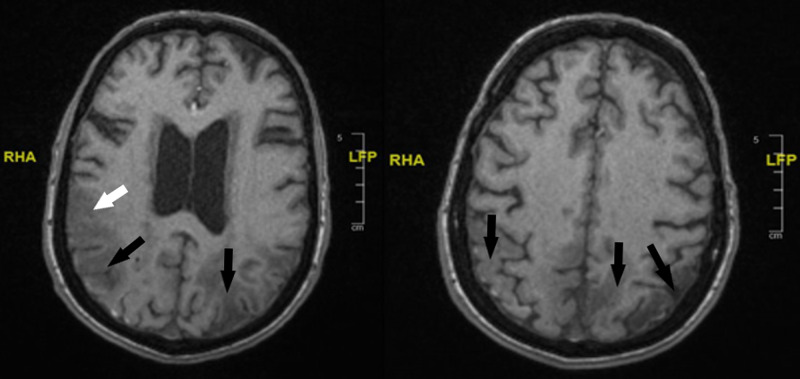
T1 axial MRI head showing widespread bright signals in the bilateral parieto-occipital regions (black arrows) and to a lesser extent involving the Temporal lobes (white arrow)
